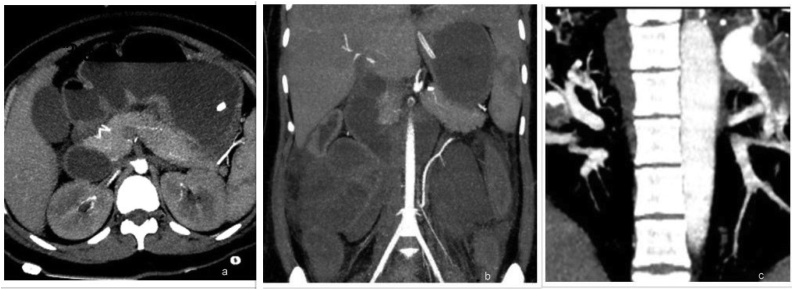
CT Angiography arterial phase images show: Completely occlusive non enhancing thrombus in superior mesenteric artery beginning at origin from aorta (Fig. 2a & b). Hypodense thrombus in Main pulmonary artery, Left and right pulmonary arteries and their branches (Fig. 2c).
